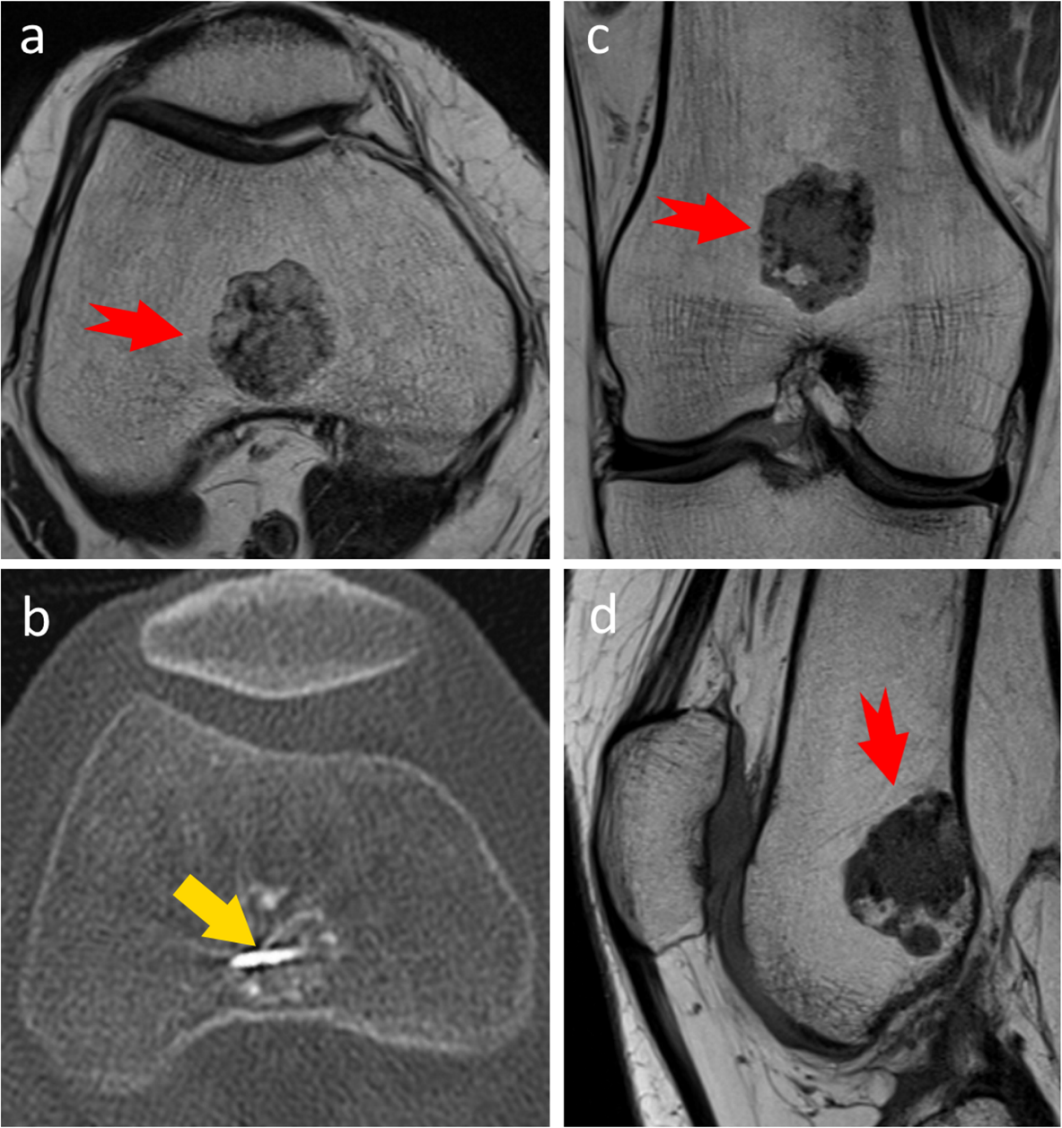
a, c, and d Preoperative magnetic resonance images in different planes to evaluate an atypical cartilaginous tumour located in the metaphysis of the distal femur, with the tumour being indicated by the red notched arrow. b An intraprocedural computed tomography image demonstrating the final location of the tip of the radiofrequency ablation electrode
